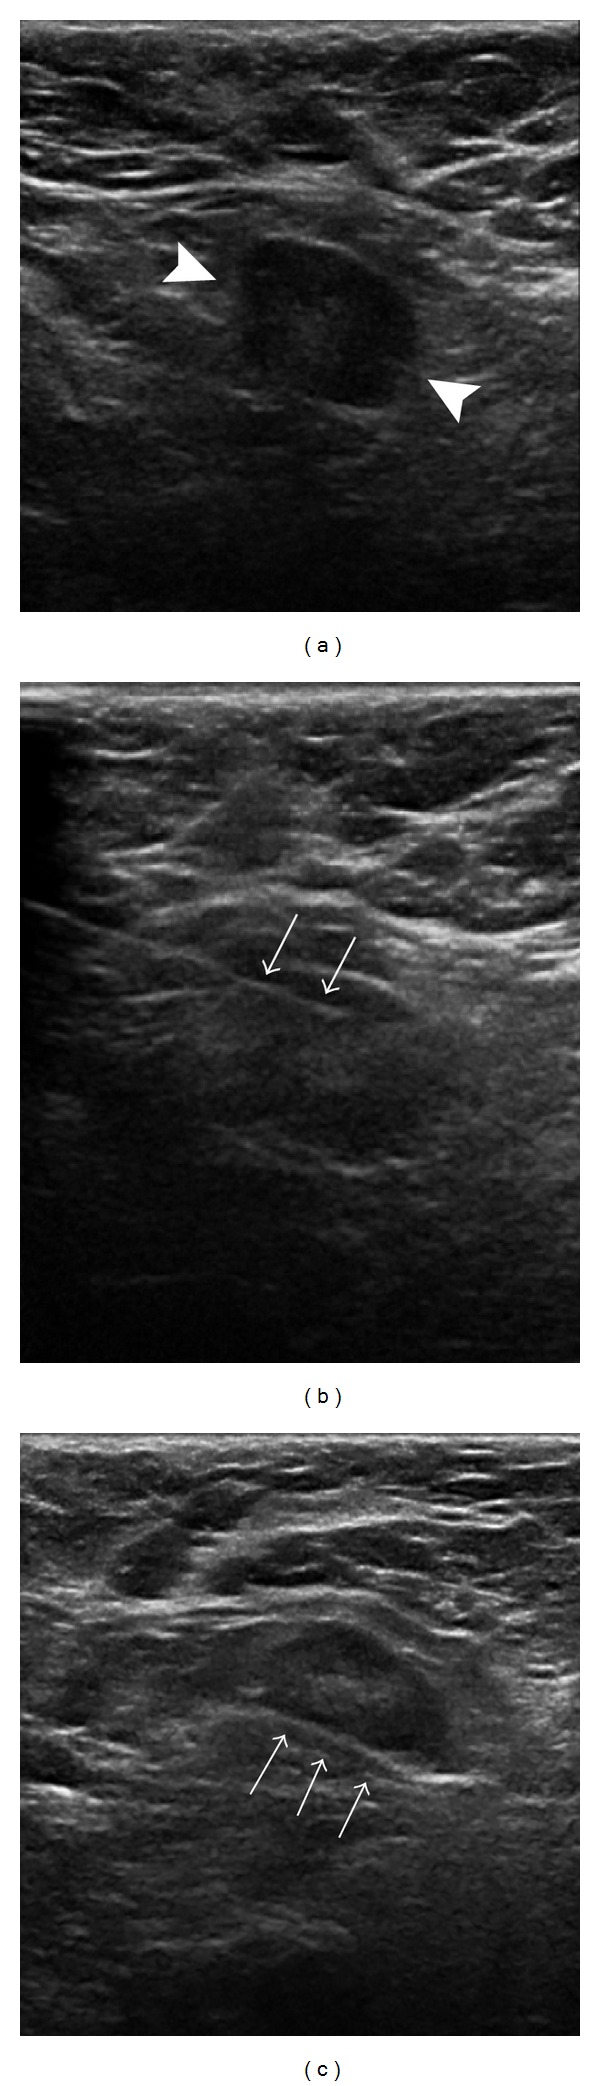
Ultrasound images of the right axilla of a 65-year-old woman with infiltrating lobular carcinoma show (a) a round lymph node (arrows) with a 5 mm cortex, (b) a 25 g FNA needle (arrows) traversing the cortex of the node, and (c) the open trough (arrows) of a 12 g core biopsy needle in the node. The FNA was single entry. The core was 1 pass. The FNA cytology was negative but the core biopsy was positive for malignancy; 7 of 18 lymph nodes were positive at axillary dissection performed less than 2 months after the biopsy.
